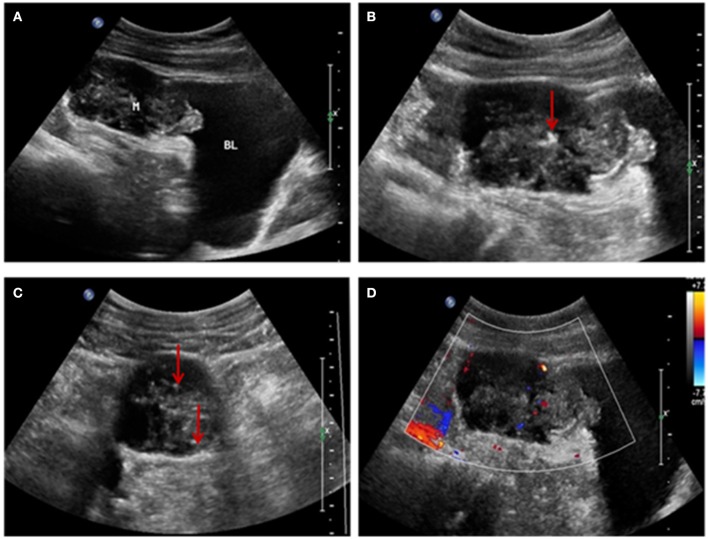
(A) Ultrasonographic image showing a solid mass above the bladder invading the bladder wall and with an irregular shape and uneven internal echogenicity. (B,C) Patchy calcification within the mass (red arrow). (D) Color-flow Doppler image showing patchy and short-line blood flow signals within the mass.
